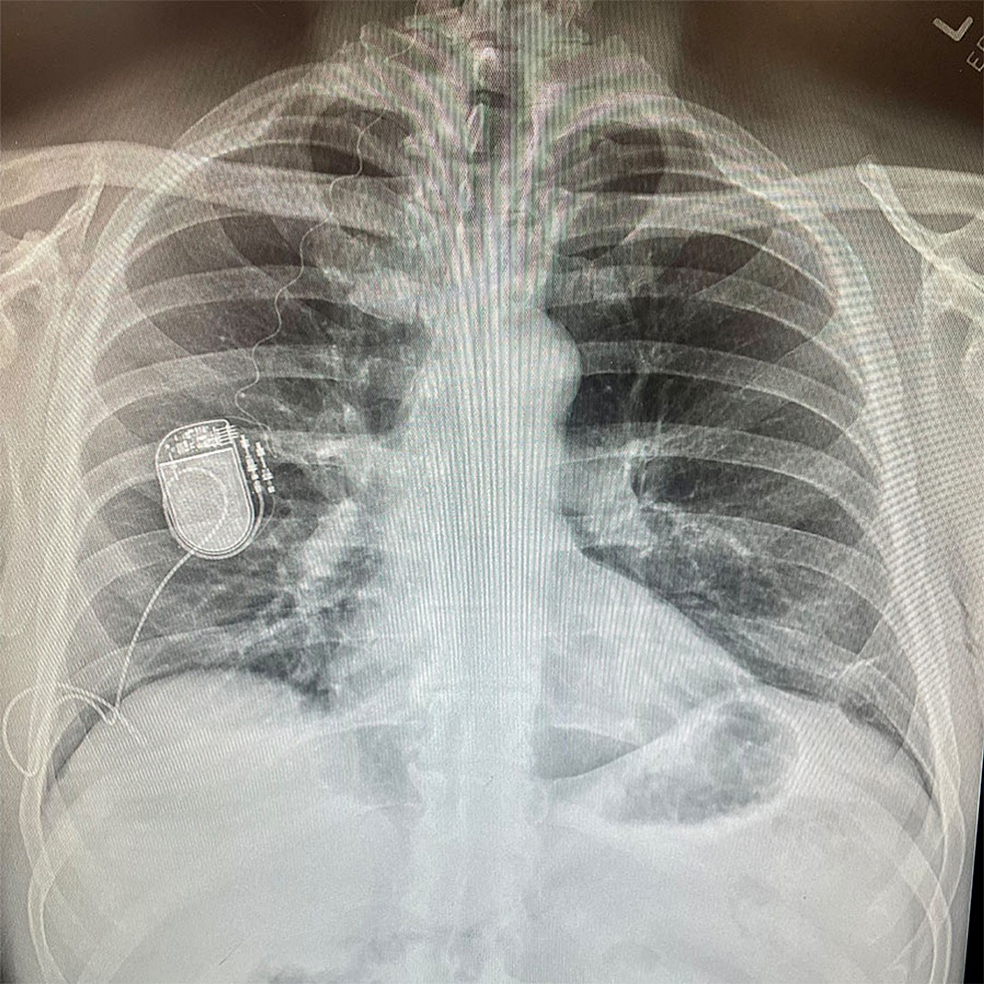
Chest radiograph showing the HGNS system. HGNS: hypoglossal nerve stimulation.
